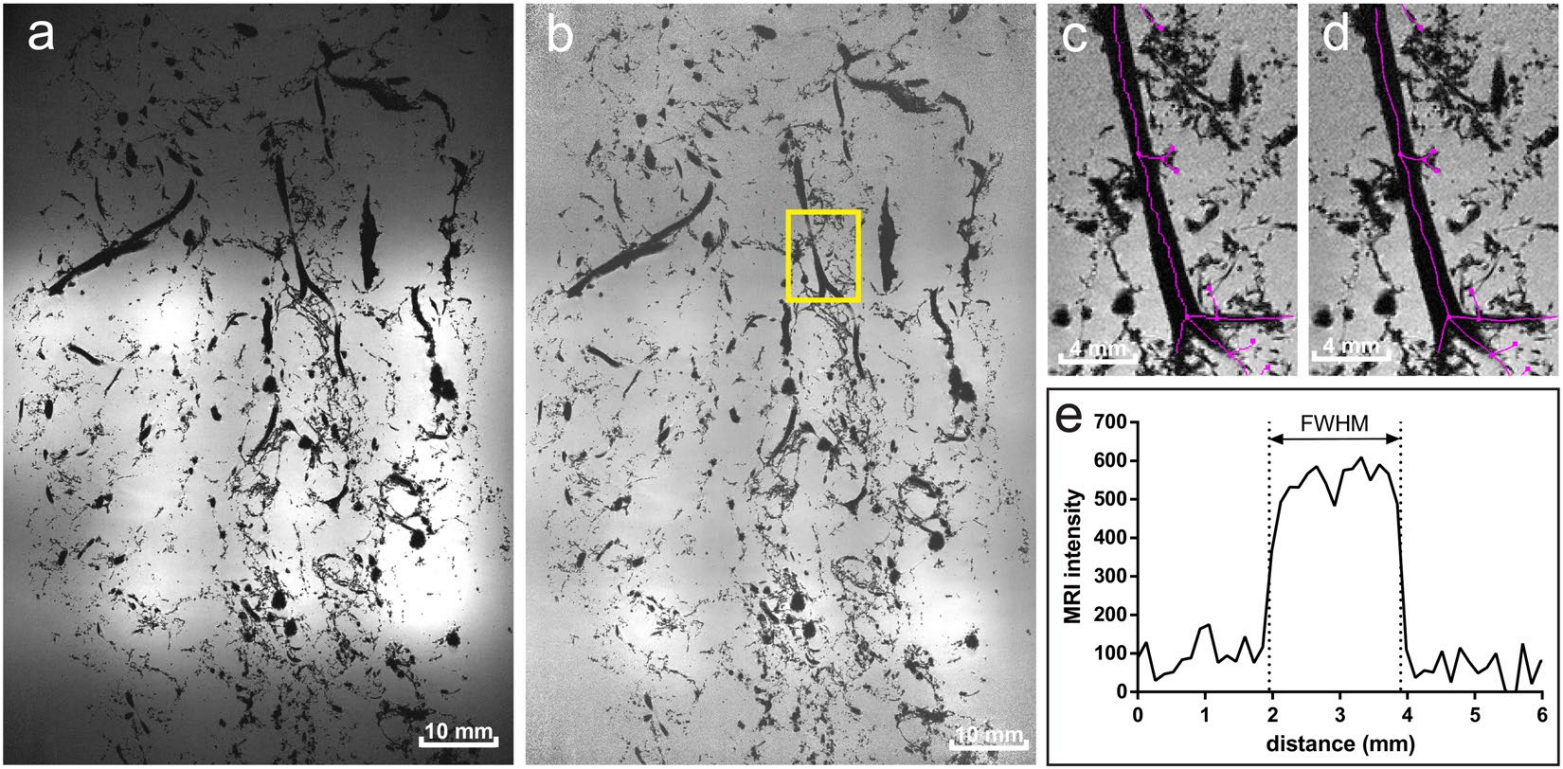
Post-processing steps. (a) Original 7T MRI single image showing the signal inhomogeneity due to local small receive coils. (b) Normalized image with yellow square marking close-up region of interest. (c) Close-up of non-smoothed centerlines. (d) Close-up of smoothed centerlines. (e) Example of one intensity profile trough the artery on which the estimated radius (obtained with full-width at half-maximum method) of the artery is depicted.
